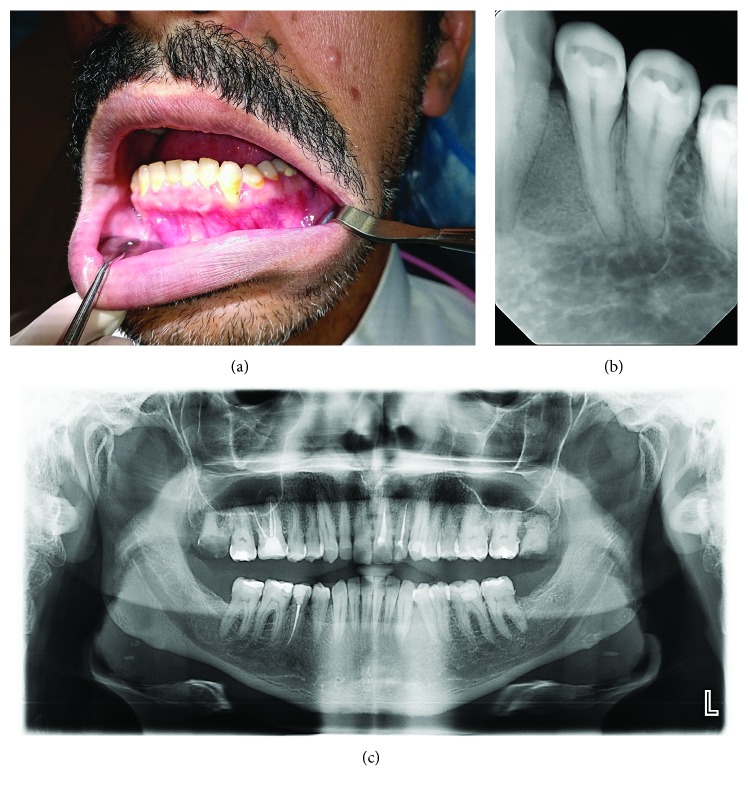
Postoperative clinical photograph and radiographs at one-year follow-up showing (a) healthy gingiva and periodontium in between teeth 33 and 34; (b) periapical radiograph and (c) orthopantomograph showing no evidence of cyst recurrence and complete bone fill between roots of teeth 33 and 34.
